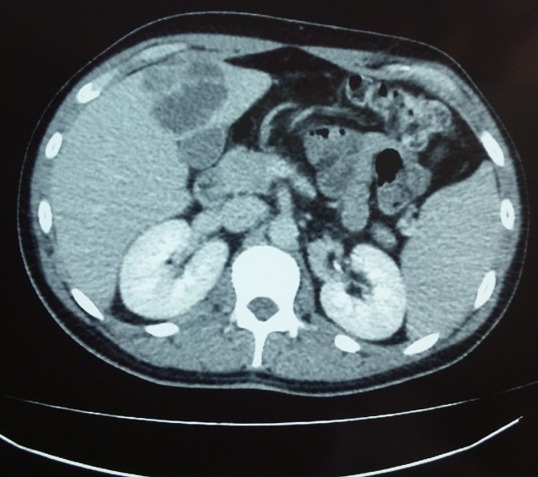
abdominal computed tomography (CT) showing an abscess in the left hepatic lobe
